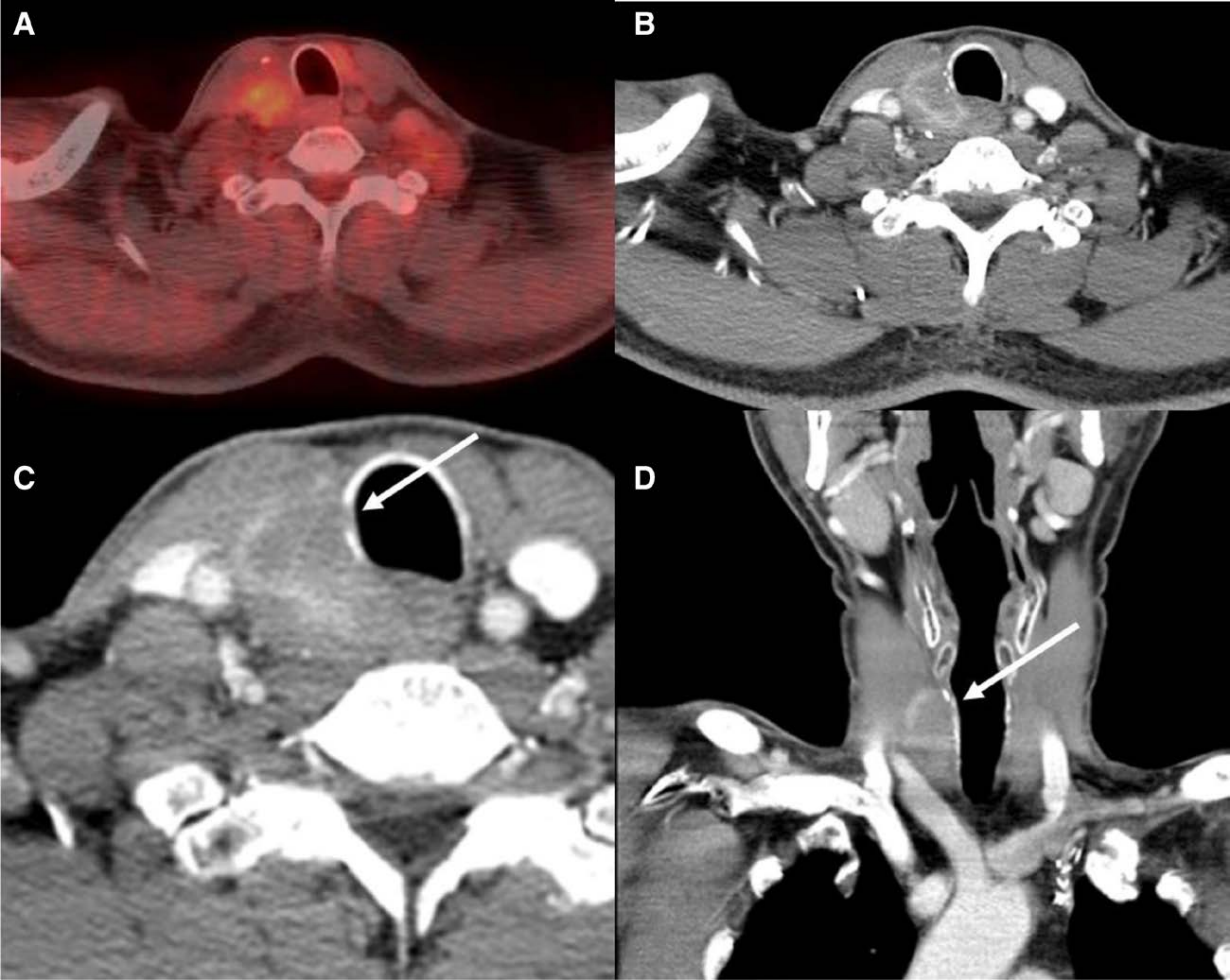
Preoperative imaging studies. (A) On 18^F^-fluorodeoxyglucose positron emission tomography-computed tomography, a right thyroid operative bed lesion showed heterogeneous hypermetabolism. (B) An axial computed tomography image showed an ill-defined hypo-enhancing mass at the right thyroid operative bed. (C) Axial and (D) coronal images showed focal decreased density at the right tracheal cartilage (arrow).
